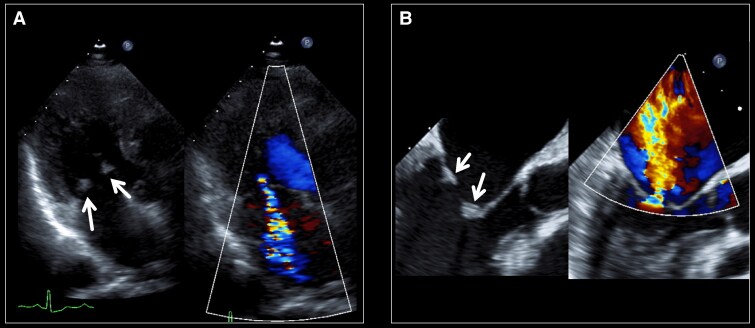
Transthoracic (*A*) and transoesophageal (*B*) echocardiography in February 2019 showing non-bacterial thrombotic endocarditis (white arrow) and severe mitral regurgitation.
